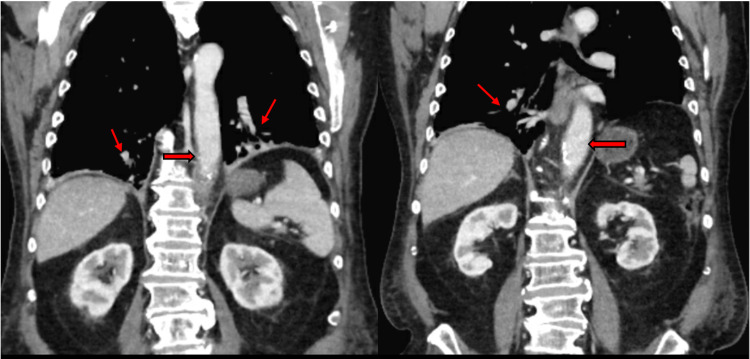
CT of the chest, abdomen, and pelvis with contrast revealed bibasilar parenchymal consolidation (oblique red arrows) and mild dilation of the proximal small bowel with no evidence of acute pulmonary embolism or aortic dissection (horizontal red arrows).
